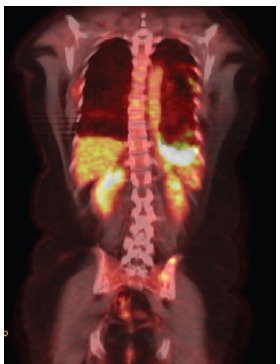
PET–CT after erlotinibe with reduction of thickening in the left pleural and increase on the FDG capture.
